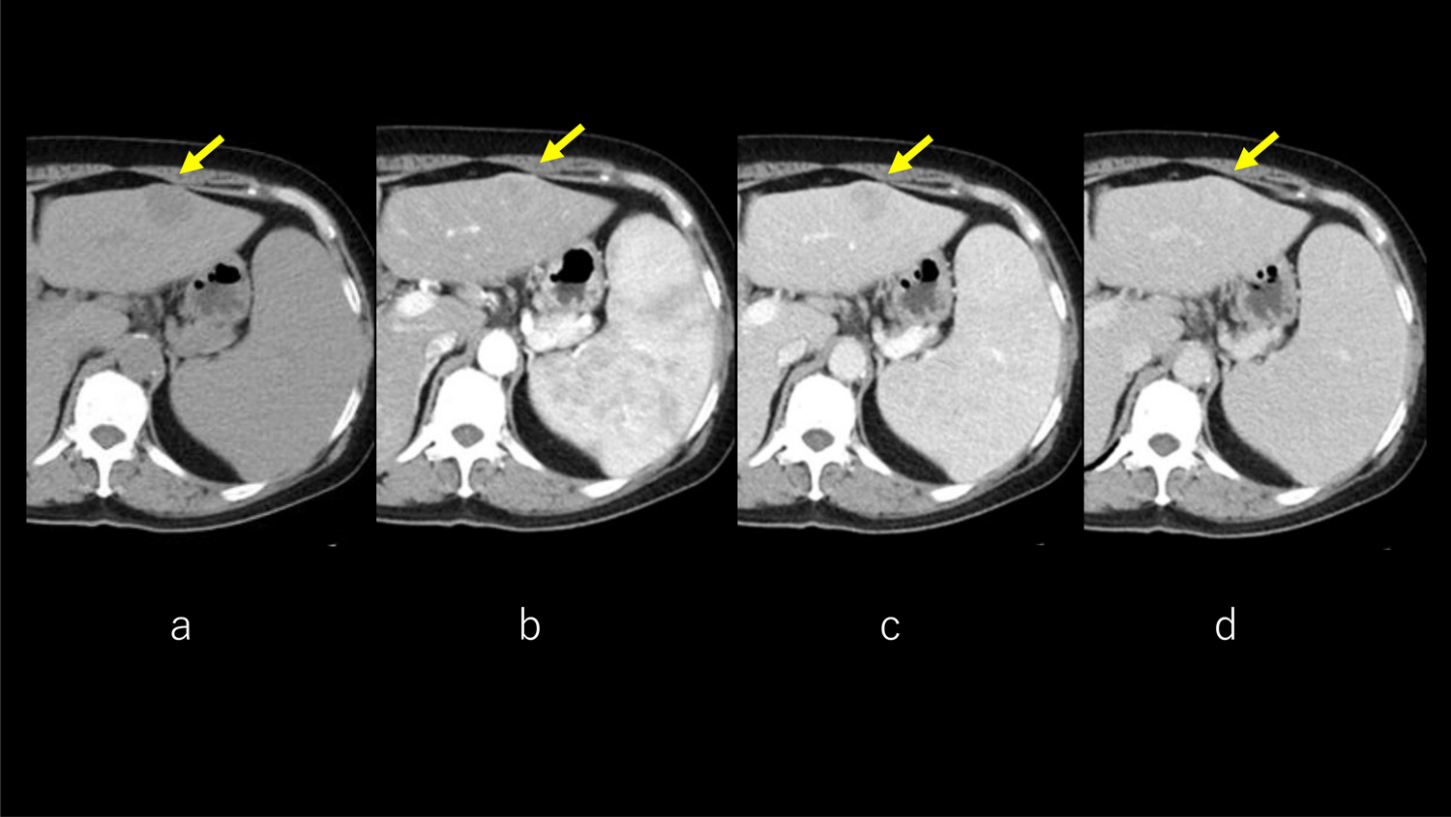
CT. Abdominal plain CT shows solitary, hypoattenuating tumor (arrow) in the left lobe (A). The arterial phase of dynamic CT shows tumor enhancement with central part sparing (B). The tumor is demonstrated clearly in the portal phase (C). The equilibrium phase of dynamic CT shows the lesion with persistent enhancement (D). CT, computed tomography.
